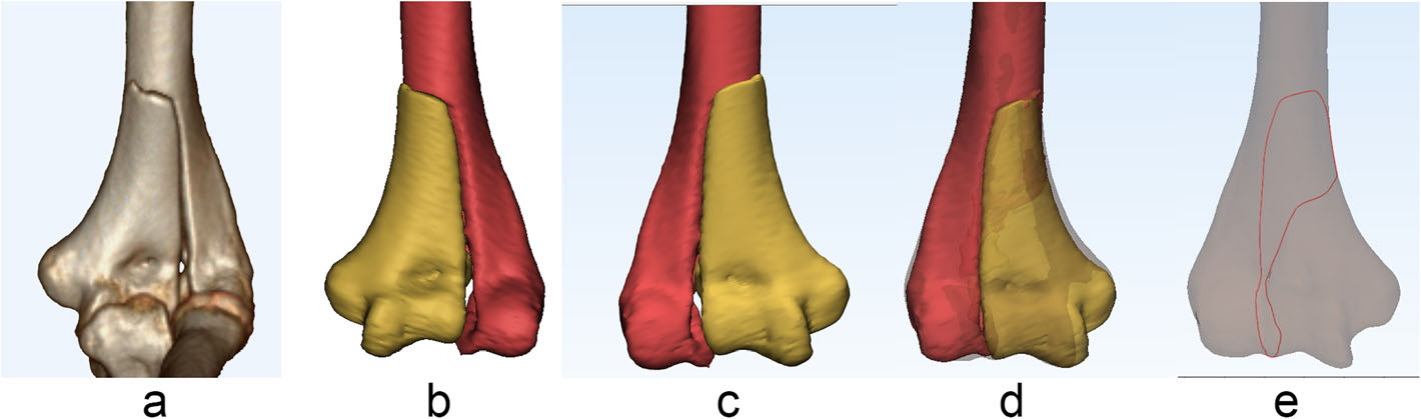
Mapping method of distal humerus fractures. a 3D image of distal humerus fracture in CT scans. b Major fragments were reconstructed in Mimics. c Model of left-sided humerus was mirrored to make the orientation match that of the right-sided humerus in 3-Matic. d The reconstructed fragments were reduced, moved, rotated, and normalized to best match the normal model of the distal humerus. e Fracture lines were delineated on the template
